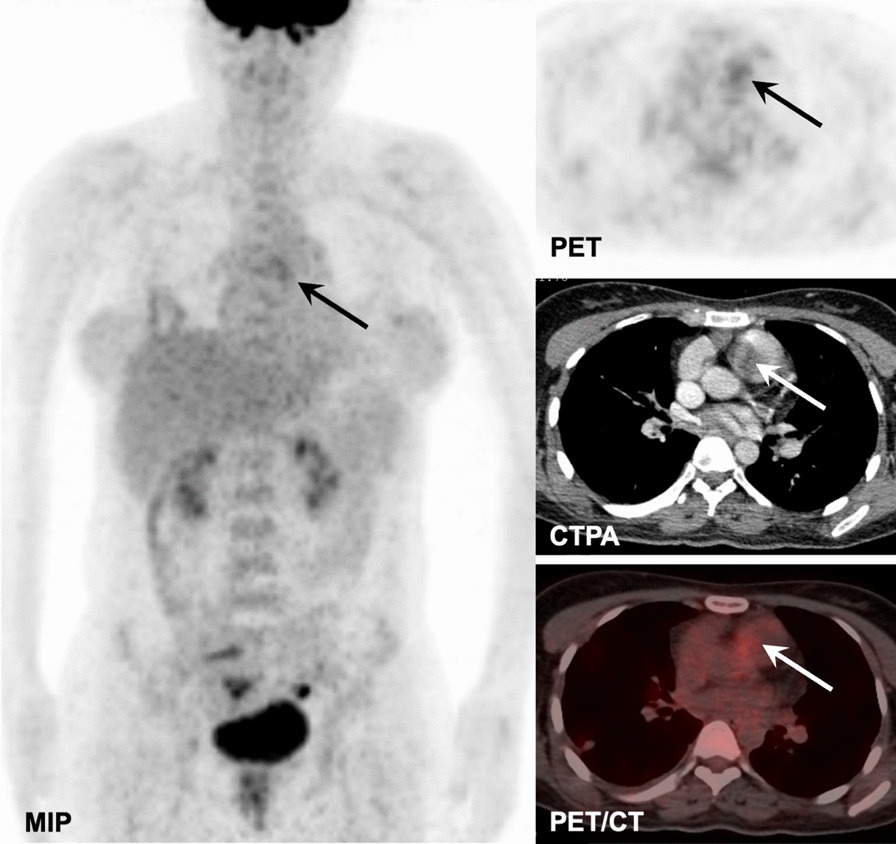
PAS in a 37-year-old woman involving PT and RPA (solid arrows) with moderate FDG uptake (SUVmax: 3.0) on the MIP, axial PET, and fused PET/CT images, and filling defect on the CTPA image
